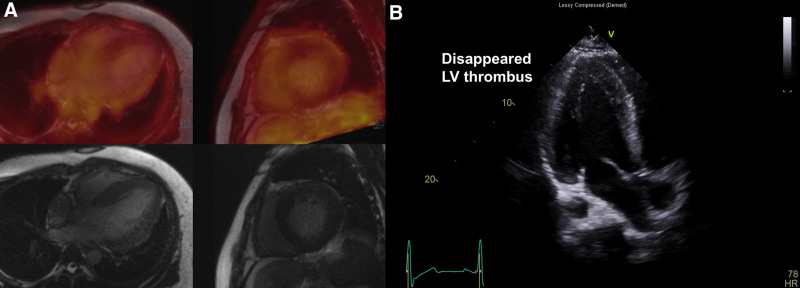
Postprocedural patient evaluation. (A) Hybrid FDG-PET/MR showing no abnormal FDG uptake or LGE, and (B) follow-up echocardiography showing resolution of the LV thrombus. FDG = 18-fluoro-deoxyglucose, LGE = late gadolinium enhancement, LV = left ventricle, PET/MR = positron emission tomography/magnetic resonance.
